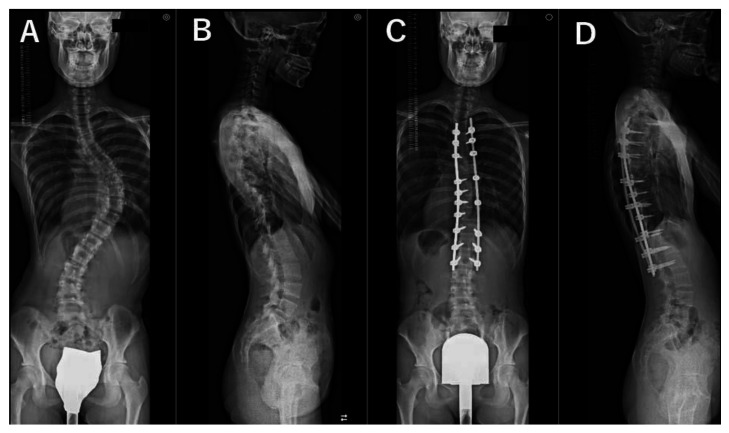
Pre- (A,B) and post- (C,D) operative radiographs of representative case with 17-year-old female patient. Cases of preoperative cervical hyperkyphosis changed to postoperative cervical kyphosis. Cervical lordosis (−19°, −8°), thoracic kyphosis (20°, 26°), lumbar lordosis (59°, 64°), T1 slope (11°, 12°), major thoracic curve (78°, 18°). (Preoperative, postoperative).
